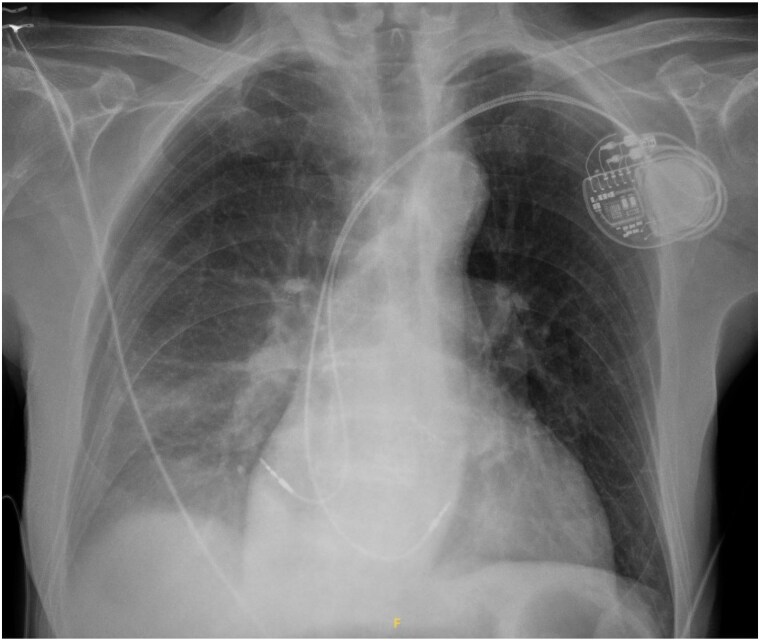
Chest radiograph obtained 6 h after pacemaker implantation demonstrating a large right-sided pleural effusion extending to the mid-lung field.
